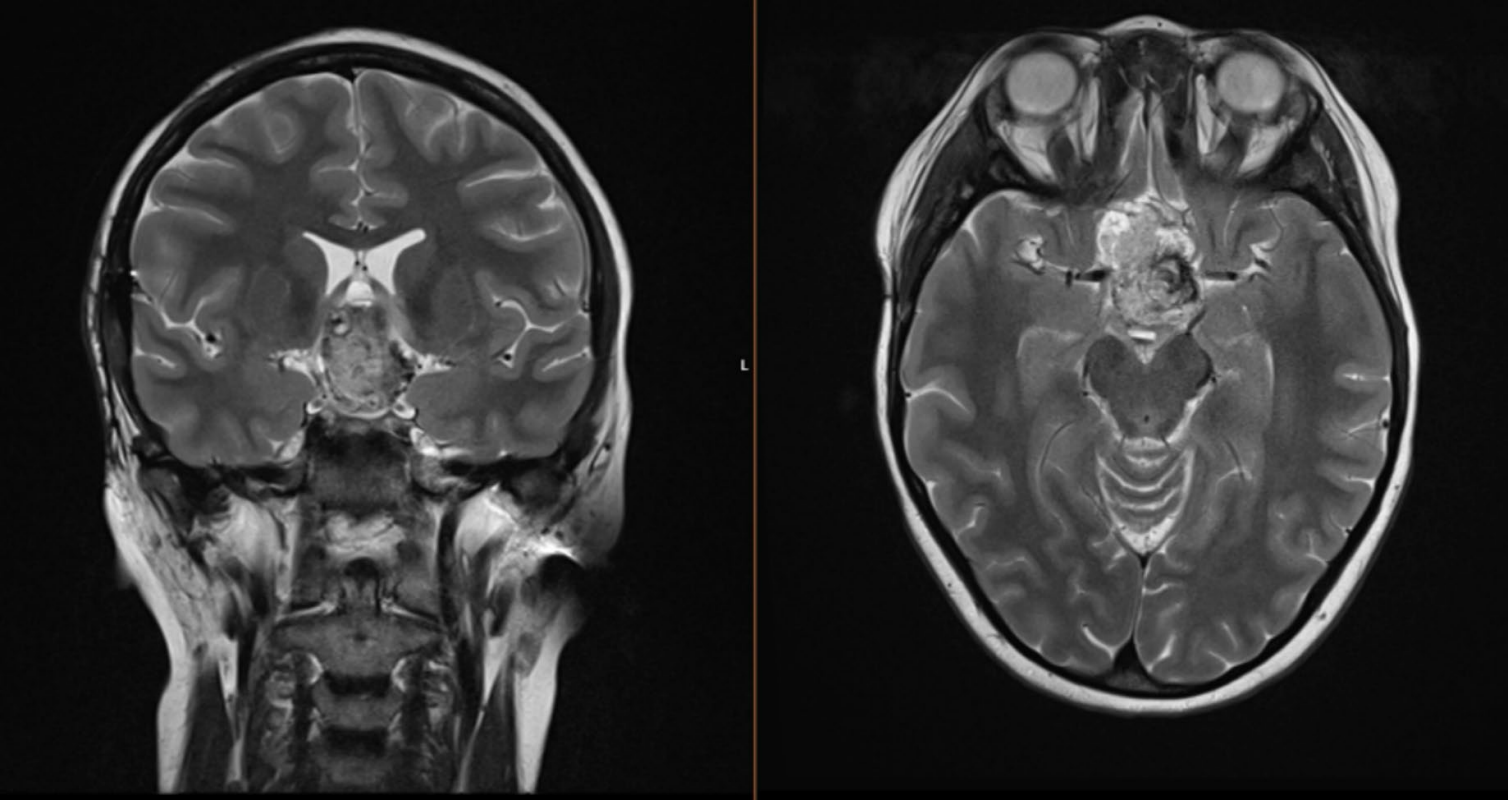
MRI brain (T2 sequence): heterogeneously T2 hyperintense suprasellar lesion measuring 26 × 43 × 29 mm (transverse × AP × craniocaudal)
